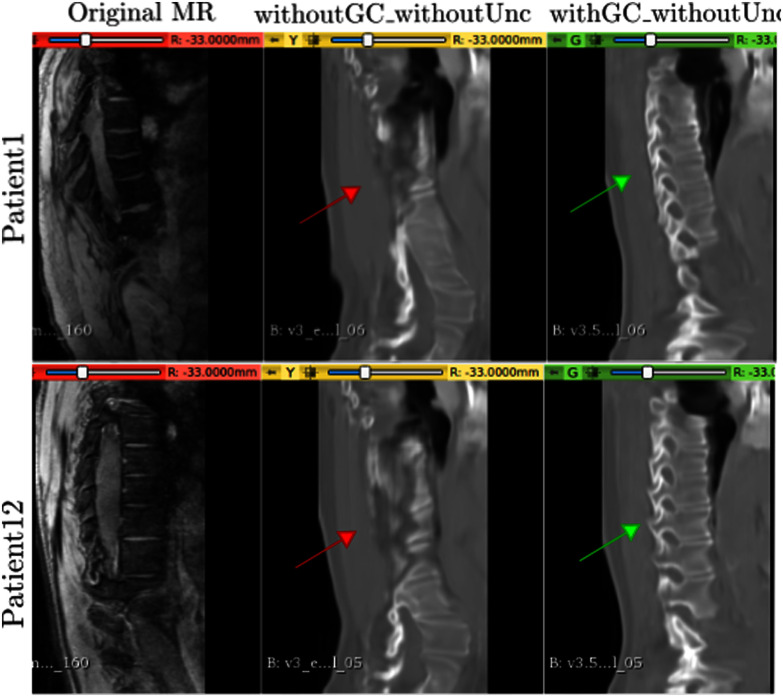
Hard Synthesized CT predictions for P1 and P12. Left-to-right: Original MR slice, synthesized CTs without uncertainty for models without and with GC, respectively. Red and green arrows show the difference in translation without and with GC. Unc.$=$ Uncertainty.
